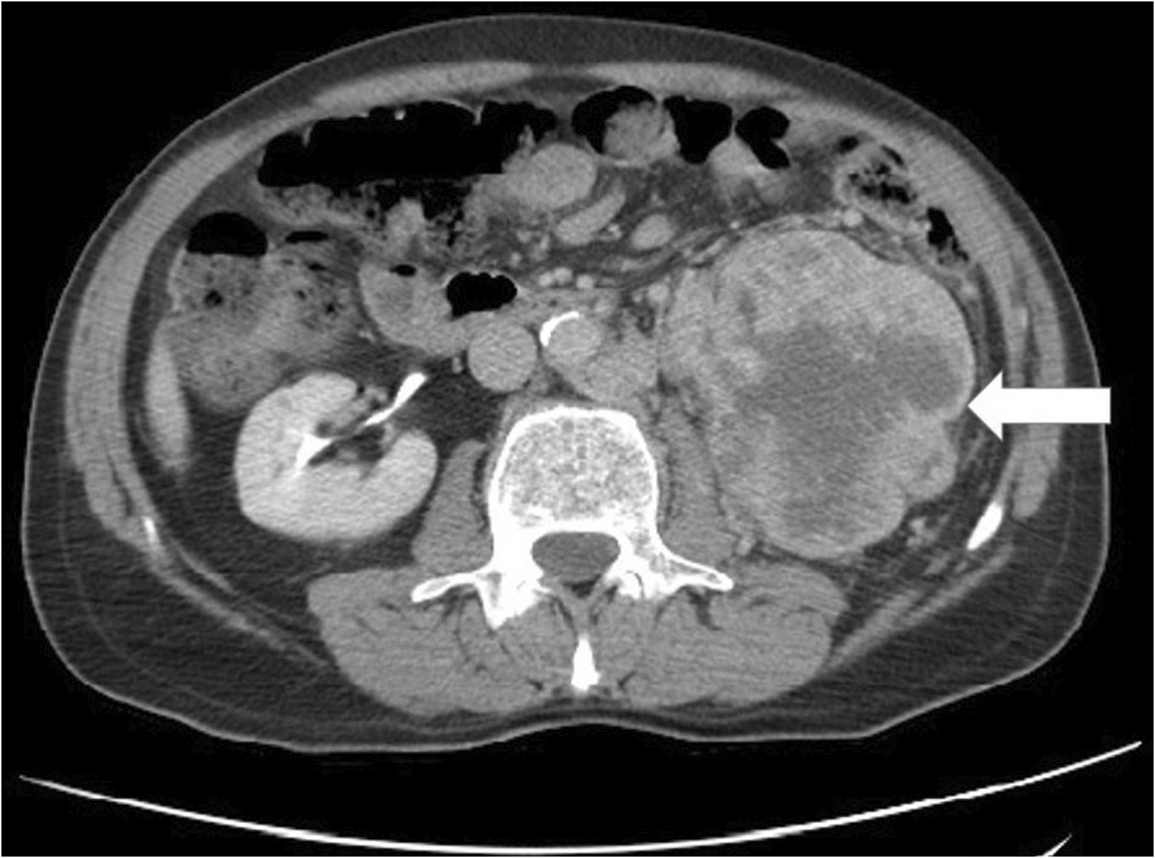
Abdominal computed tomography showed an enhancing mass lesion in left kidney
